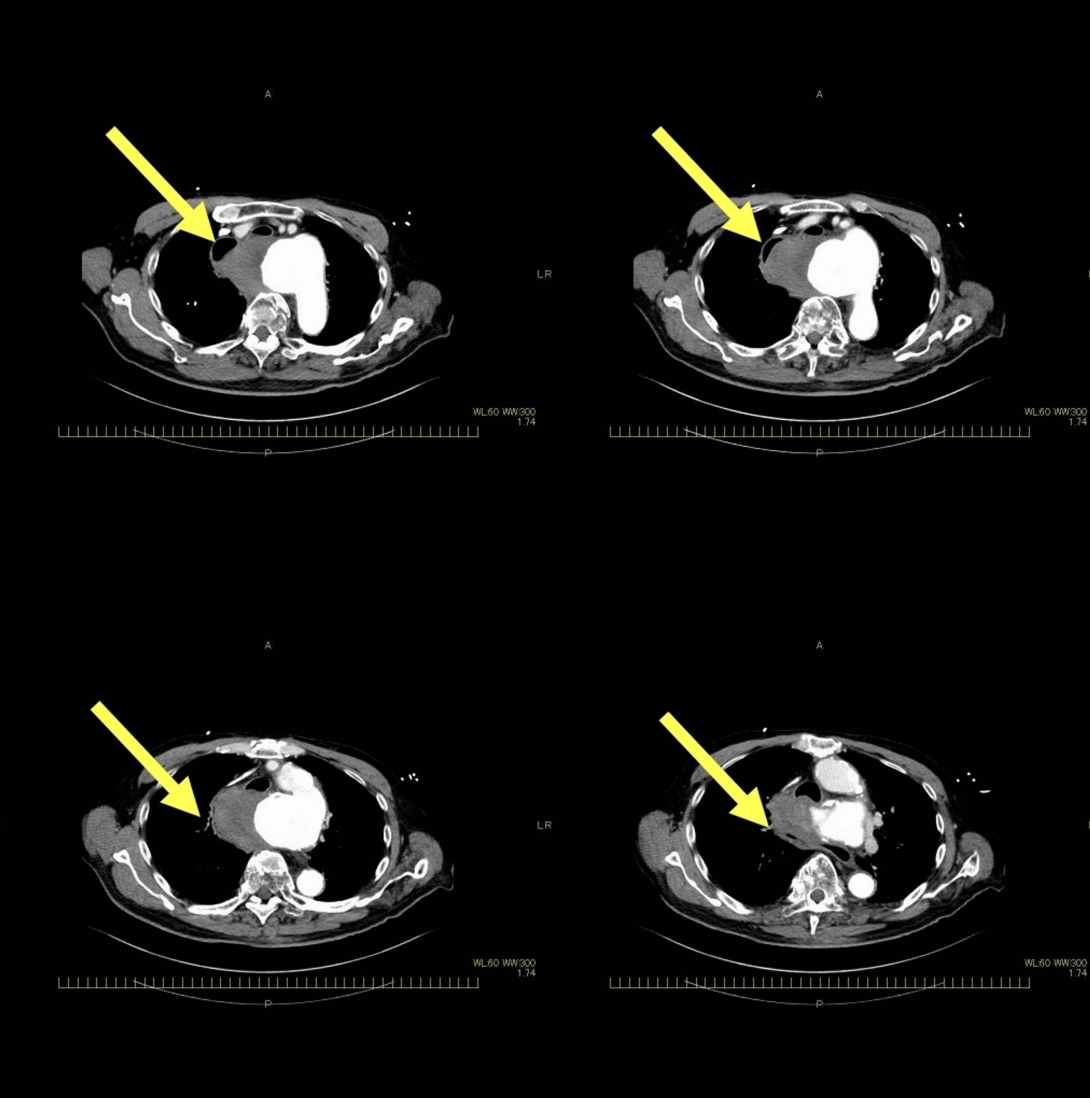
Contrast-enhanced CT Axial contrast-enhanced CT image showing a hematoma from an aortic aneurysm compressing the esophagus, suggestive of aortoesophageal fistula CT: computed tomography
